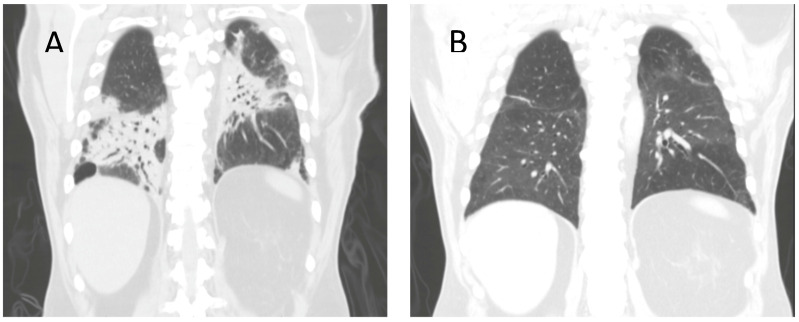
(A) CT Chest on admission showing significant multifocal airspace consolidation in mid-to-lower lung zones. (B) Repeat CT Chest after completion of steroid course, showing improved bibasilar consolidation.
